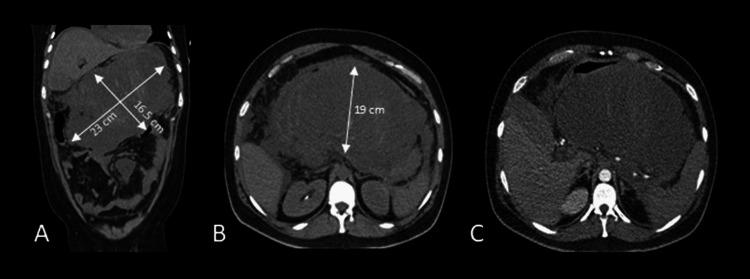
CT progression of necrotizing pancreatitis. Abdominopelvic CT scan showing disease progression, with over 80% pancreatic necrosis (CT Severity Index: 10) and a large acute necrotic collection measuring 23 × 16.5 × 19 cm, visible in coronal (A) and axial (B) planes. Angio-CT confirms active bleeding within the necrotic collection, as seen in the axial plane (C).
